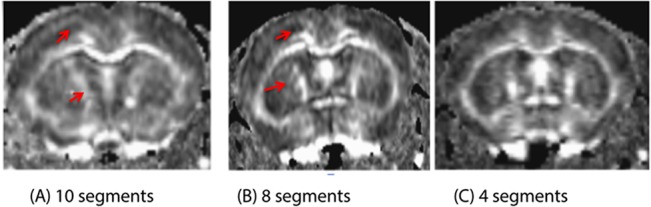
Optimization of ETL segmentation to reduce image artefacts. The FA map from 4-segment EPI-DWI (C) shows less susceptibility to motion artefacts and structure displacement compared the maps reconstructed from 10 and 8-segment ETL (A and B, respectively). This can be observed in the anterior cingulate cortex adjacent to the corpus callosum.
